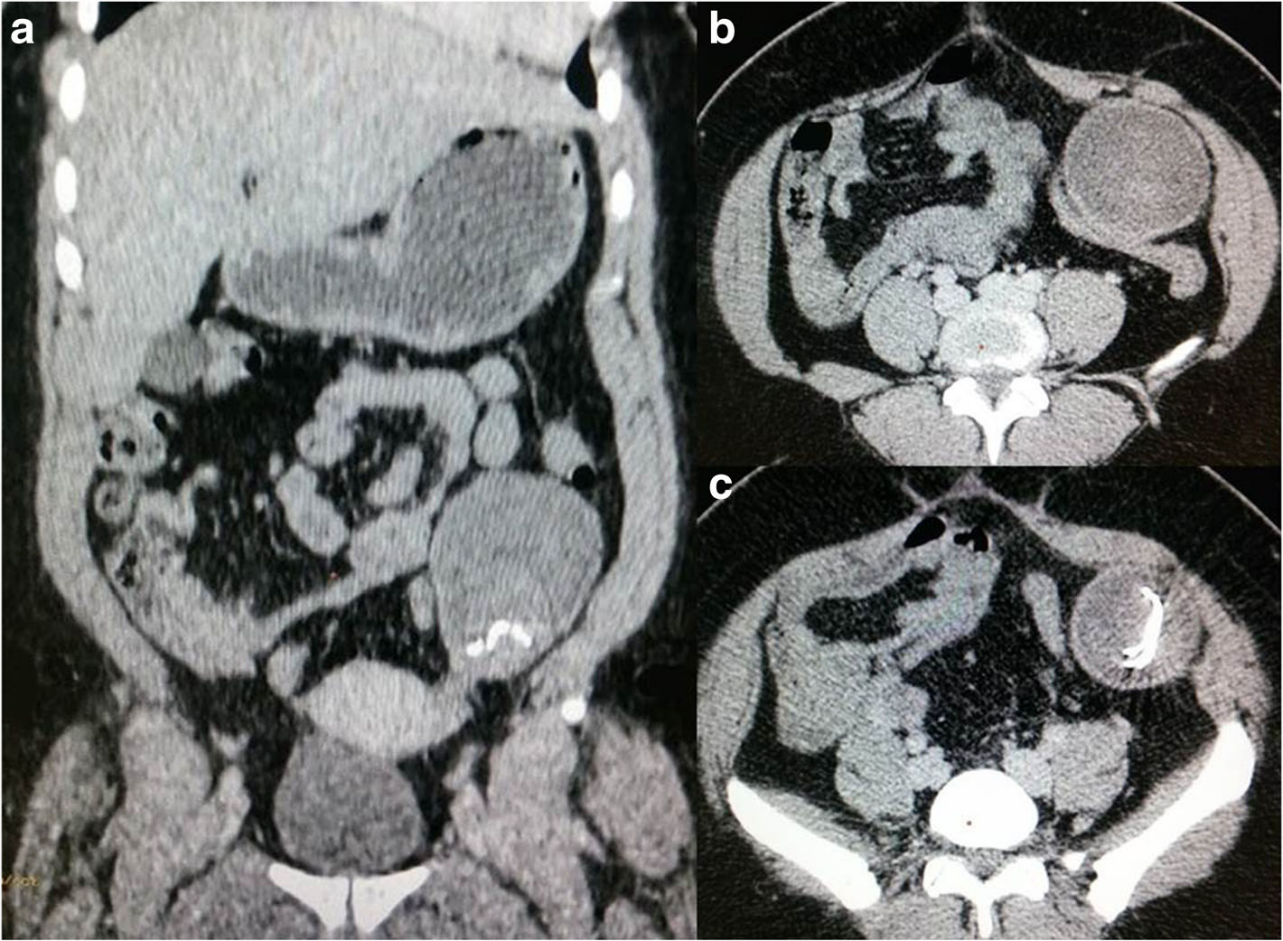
Cornal (a) and axial (b and c) abdominal CT scan images showingan organised hypodense mass (gossypiboma) in the left iliac fossa with an intern hyperdense structure corresponding to a radiopaque marker
